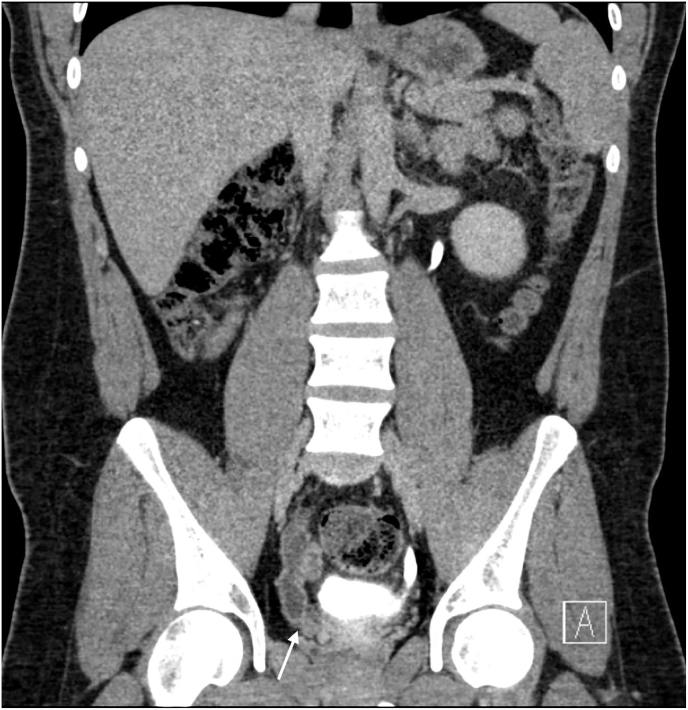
Coronal reconstructed CT urogram of the abdomen and pelvis shows a blind-ended dilated right ureter that inserts ectopically into right seminal vesicle (arrow).
